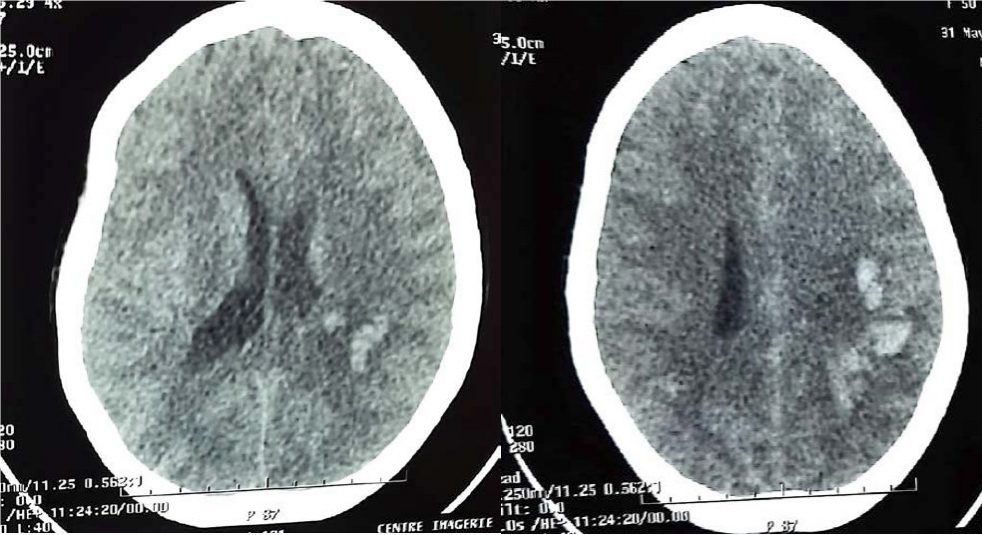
Tomodensitométrie cérébrale montrant une plage hémorragique en fronto-pariétal gauche associée à une hémorragie méningée Brain computed tomography showing bleeds in left fronto-parietal region and subarachnoid hemorrhage
